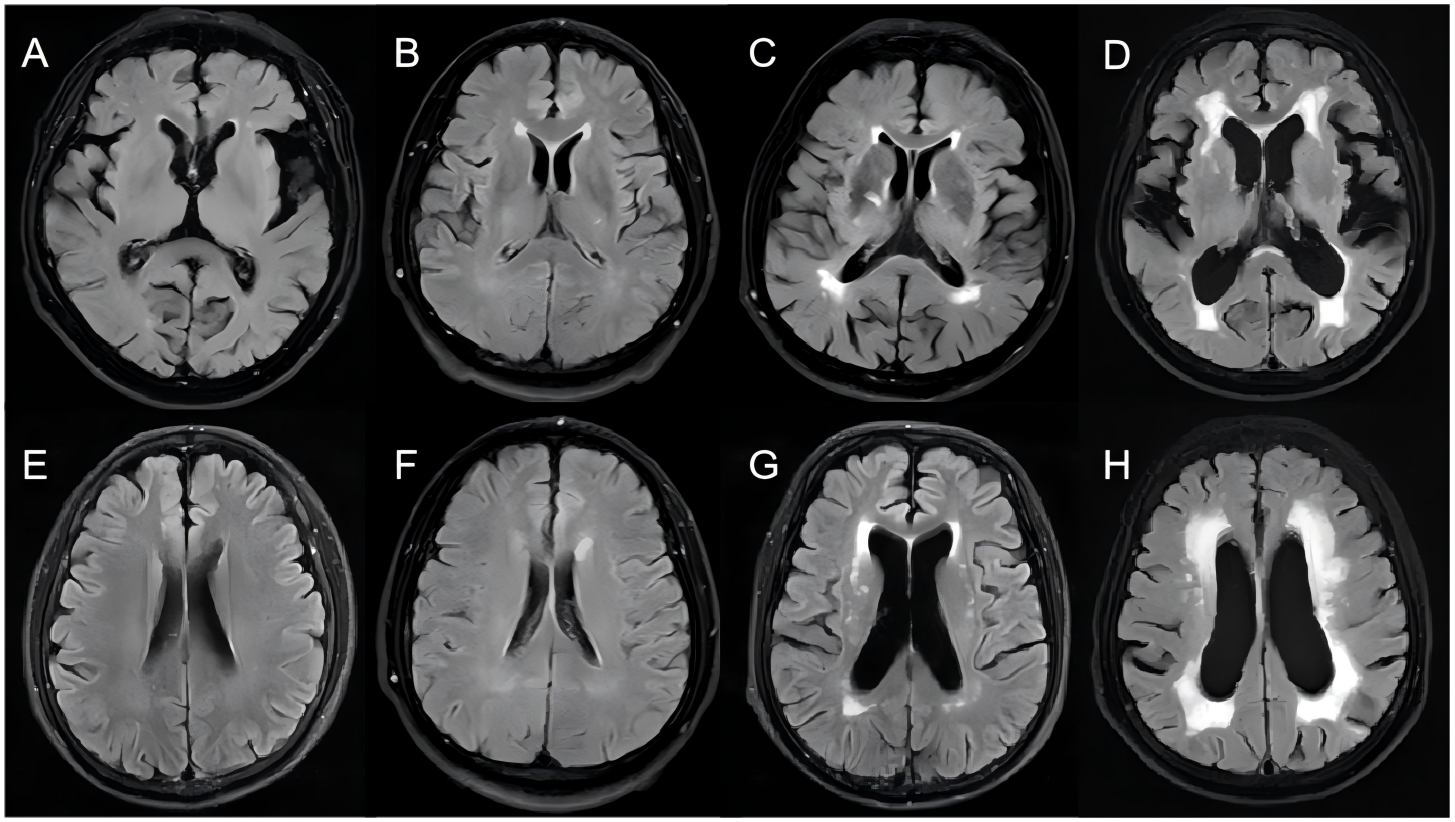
T2 FLAIR imaging reports of head MRI. Periventricular WMH (PVWMH) fazekas scores: (A) 0 points, (B) 1 point, (C) 2 points, (D) 3 points; Deep WMH (DWMH) Fazekas scores: (E) 0 points, (F) 1 point, (G) 2 points, (H) 3 points.
